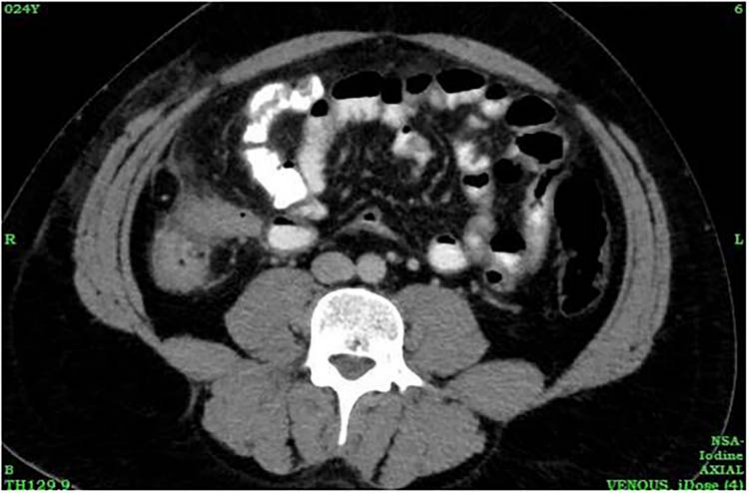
CT abdomen showing caecal wall thickening.
